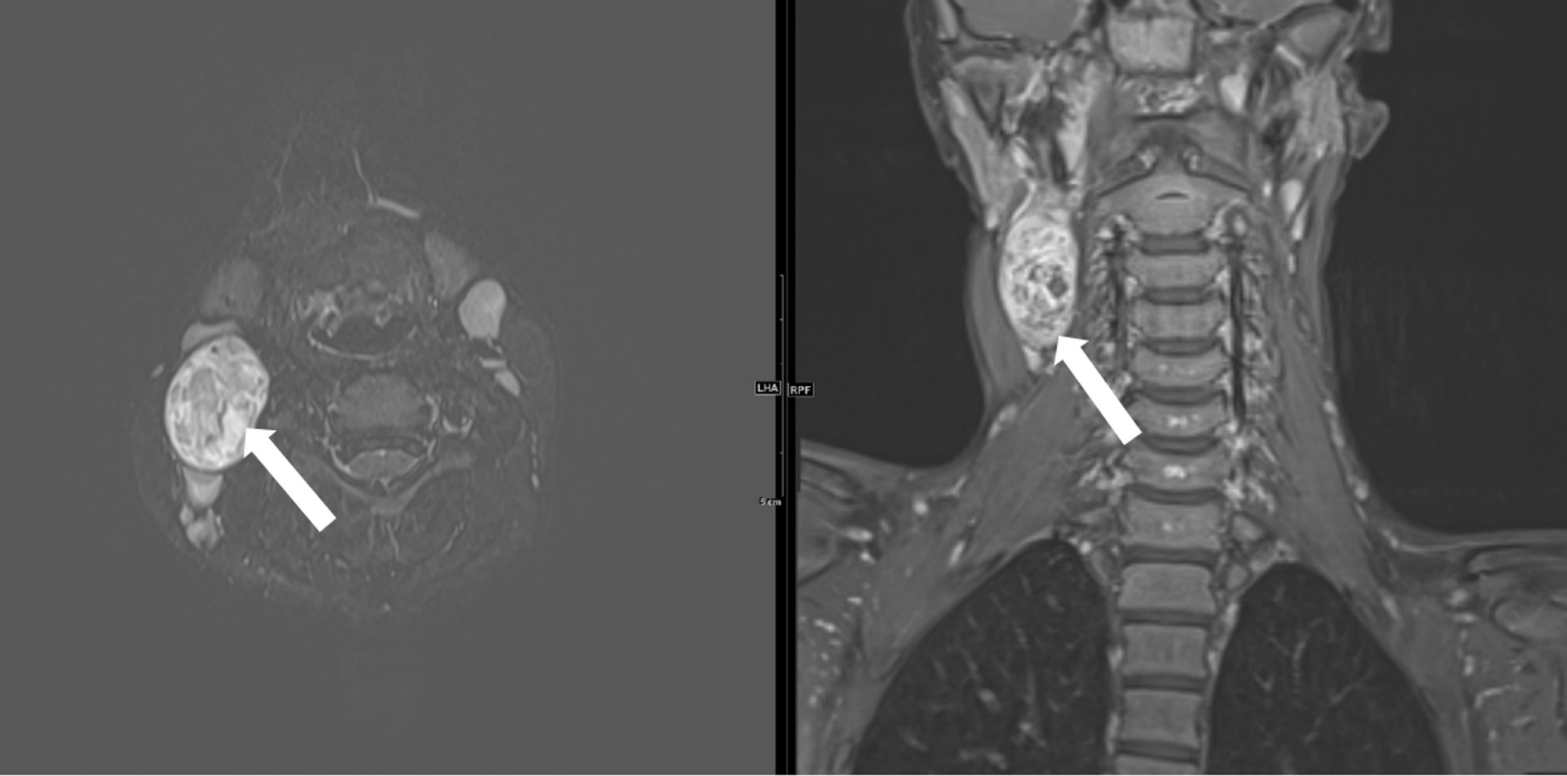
Axial T2-weighted and coronal T1-post contrast MRIs showing vagal schwannoma (arrow) with heterogeneous enhancement and non-enhancing regions suggestive of degeneration.
